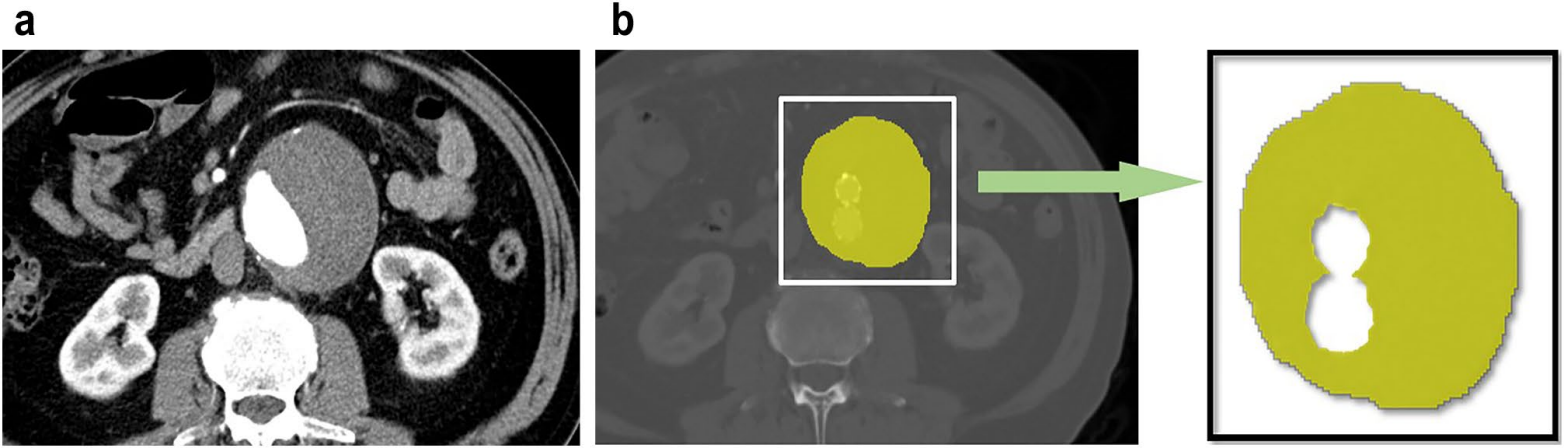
Segmentation and feature extraction process. (a) Maximal axial plane of preoperative abdominal aortic aneurysm in contrast-enhanced CT angiography. (b) Maximal axial plane of post EVAR abdominal aortic aneurysm in contrast-enhanced CT angiography. Two radiologists reviewed all the axial contrast CT scans and defined the maximum aneurysm cross section using MATLAB (R2016a, Mathworks, United States) software to manually outline the aneurysm sac area outside the stent. Abbreviation: EVAR, endovascular aneurysm repair.
